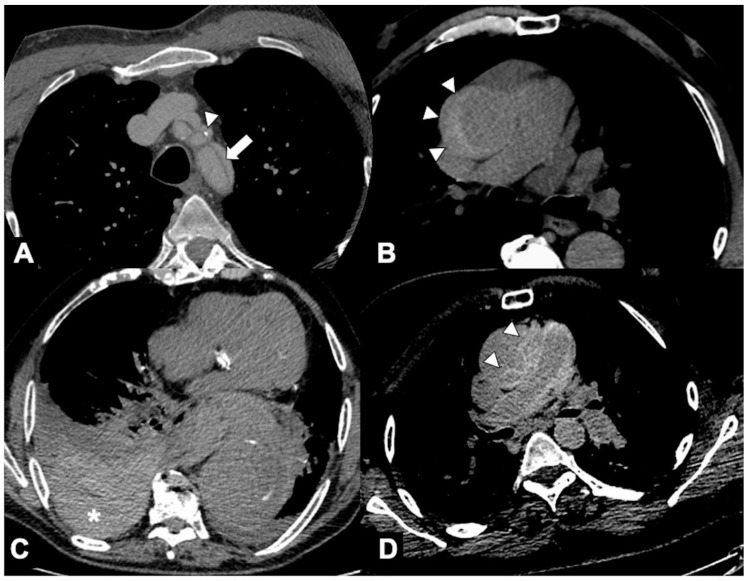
Usefulness of CTA low-dose unenhanced phase in acute aortic syndrome. (A) Noncontrast CT axial scan shows dissection flap (arrow) and displaced intimal calcifications (arrowhead) in supra-aortic vessels extension of type A aortic dissection in a 79-year-old-man with acute neck and back pain. (B) Axial image from noncontrast CT shows a crescent-shaped high-density rind in the ascending aorta, a typical appearance of type A acute intramural hematoma (arrowheads). (C) Noncontrast CT axial scan shows a large bilateral pleural effusion with increased density in the dependent aspect of the collection (hematocrit sign), highly suggestive of hemothorax (asterisk) from rupture of the descending thoracic aorta aneurysm. (D) Axial image of aorta from noncontrast CT shows hemomediastinum tracking along the main and right pulmonary arteries and hyperattenuating dissection flap (arrowheads) in ascending aorta in a 68-year-old woman with ruptured type A dissection.
